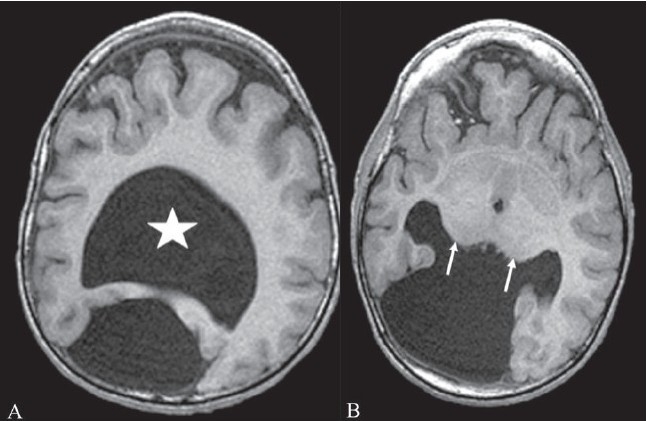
Pancake brain. Axial T1W MRI images (A, B) of the brain in alobar holoprosencephaly show fusion of the cerebral hemispheres, with a single ventricular cavity (star) in the center; this gives the brain the appearance of a pancake. Note the fusion of the thalami (arrows)
